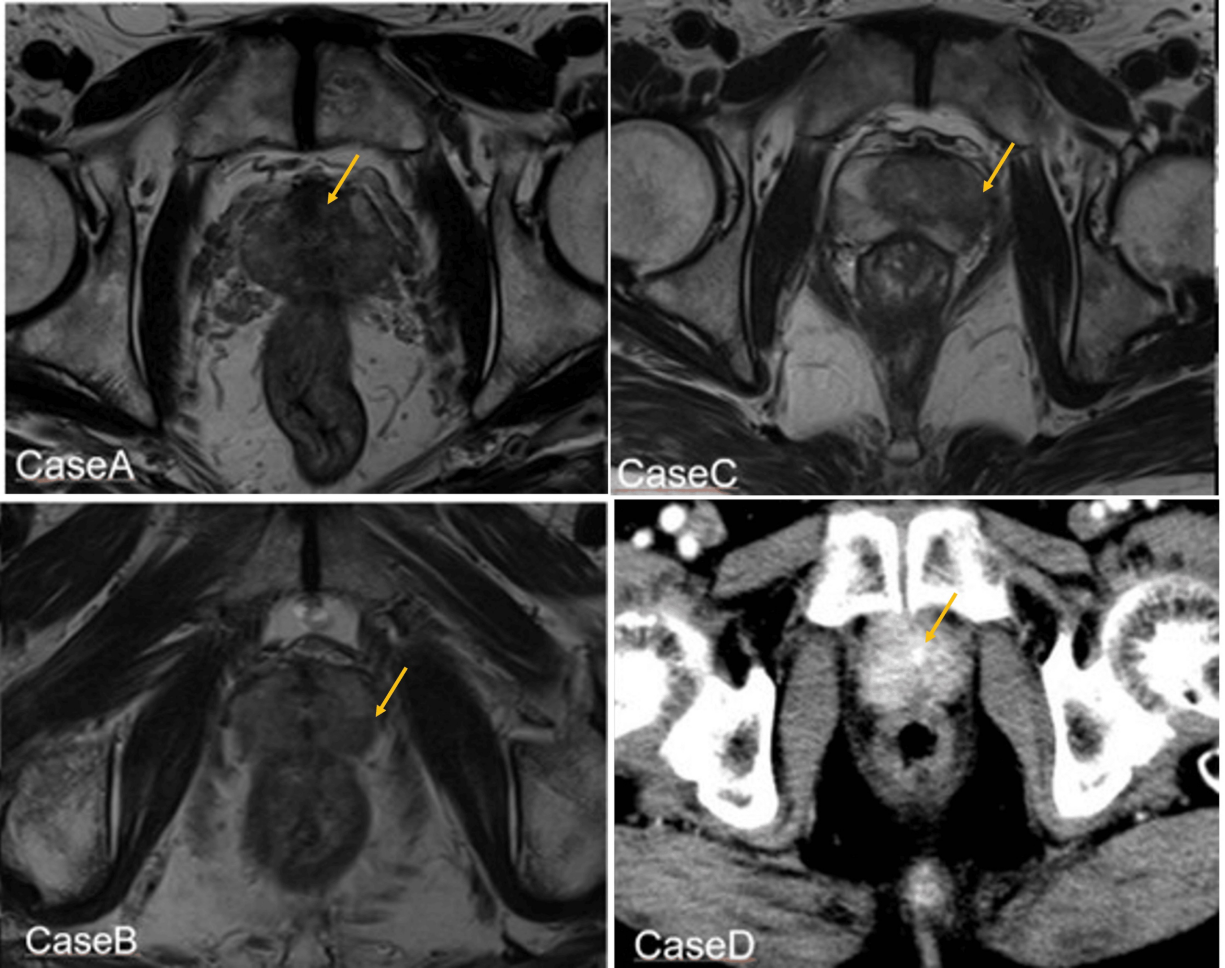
MRI images (axial) of Cases A, B, and C and contrast-enhanced CT image (axial) of Case D Prostate cancer is indicated by orange arrows. Cases A-C: At the site indicated by the arrow, T2-weighted imaging reveals an area of low signal intensity distinct from the normal structure. Case D: Contrast-enhanced CT revealed an enhancement effect in the right marginal area. CT, computed tomography; MRI, magnetic resonance imaging.
